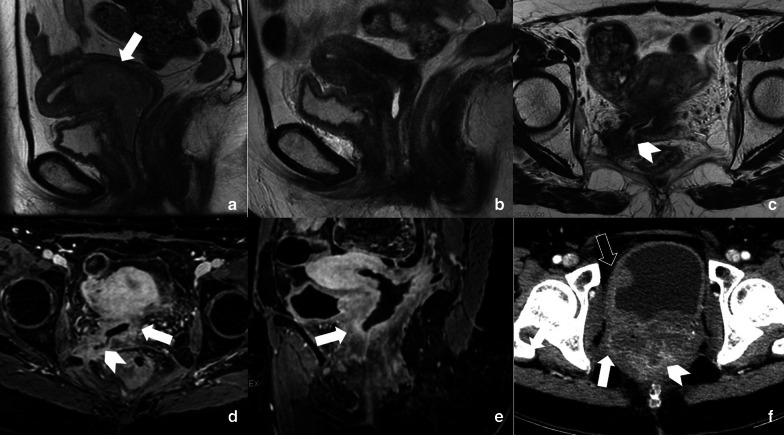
A 52-year-old woman with a FIGO IIa cervical cancer (arrow in a) treated with external beam RT and ChT, followed by high dose rate brachytherapy boost in July 2017. In December 2017 (b) sagittal T2-weighted image showed an almost normal reconstitution of hypointense cervical stroma. Axial T2-weighted MRI follow-up in April 2018 showed a fistulous tract (arrowhead in c) of the right-posterior vaginal wall at the level of fornix, that blinded-ended in correspondence of piriformis muscle. Axial post-contrast fat-suppressed T1-weighted images (d, e) showed better the fistulous tract (arrowhead in d) and irregular enhanced thickening of vaginal wall (arrows in d, e), extended to cervix, which raised a strong suspicion of disease recurrence. Axial CT image performed on September 2018 showed progressive disease since the pathologic tissue infiltrated pelvic side wall, rectum and bladder (respectively arrow, arrowhead and empty arrow in f)
